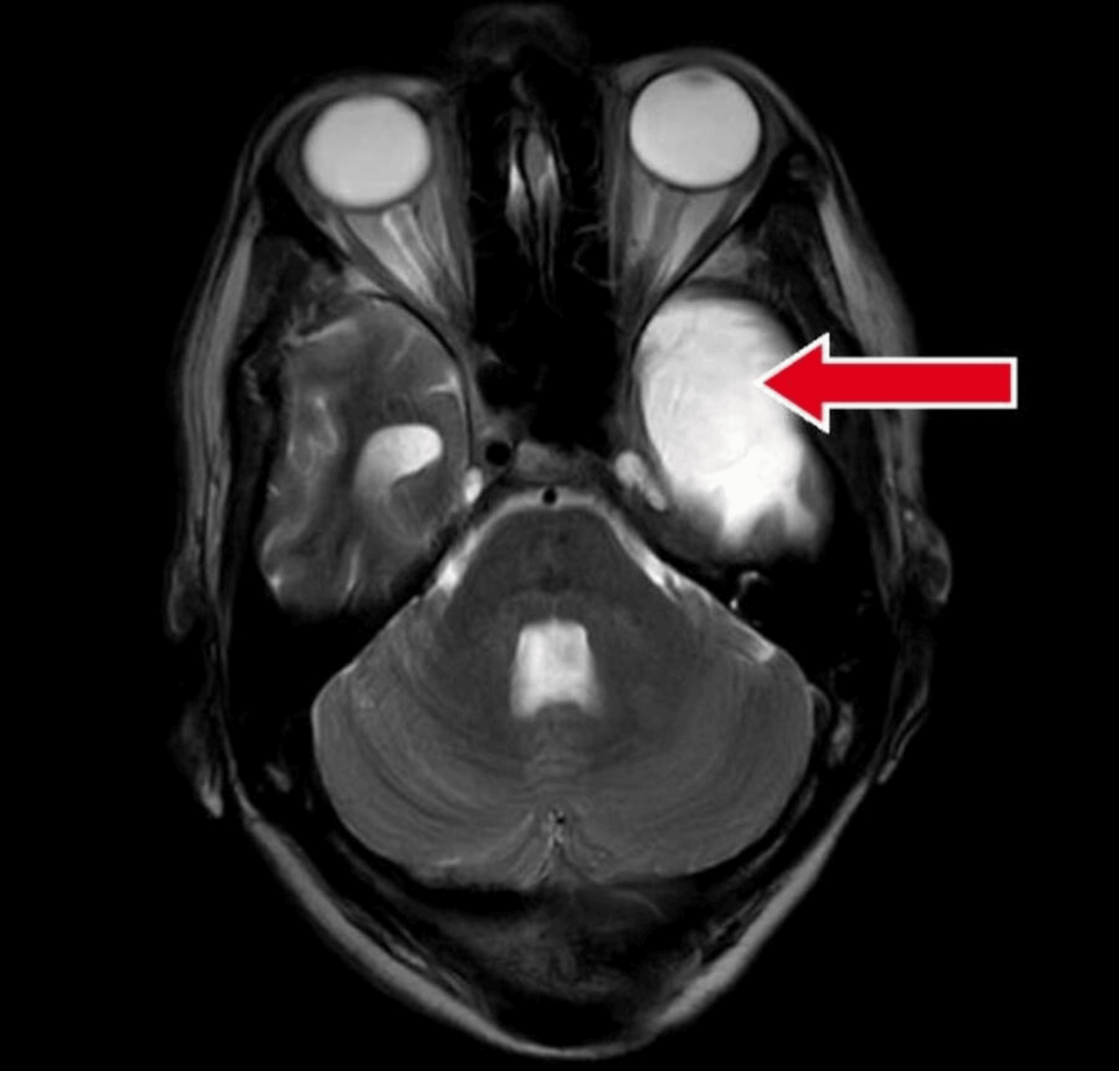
Contrast enhanced magnetic resonance imaging showing lesion in temporal lobe with red arrow.
